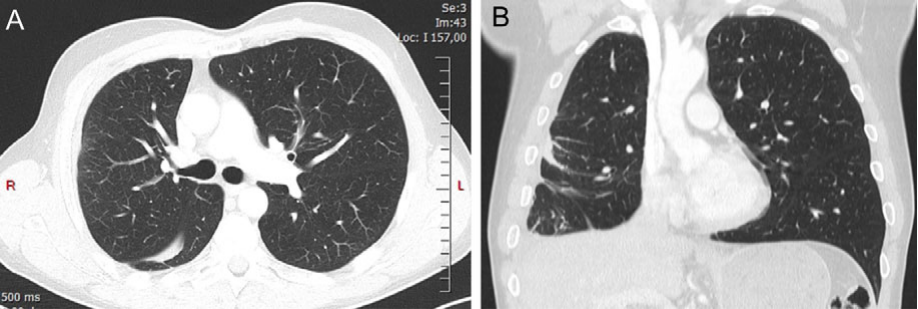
High-resolution CT scan showing right pleural thickening (A). Coronal view clearly shows both right lung volume loss and intra-lobar pleural abnormalities (B).
